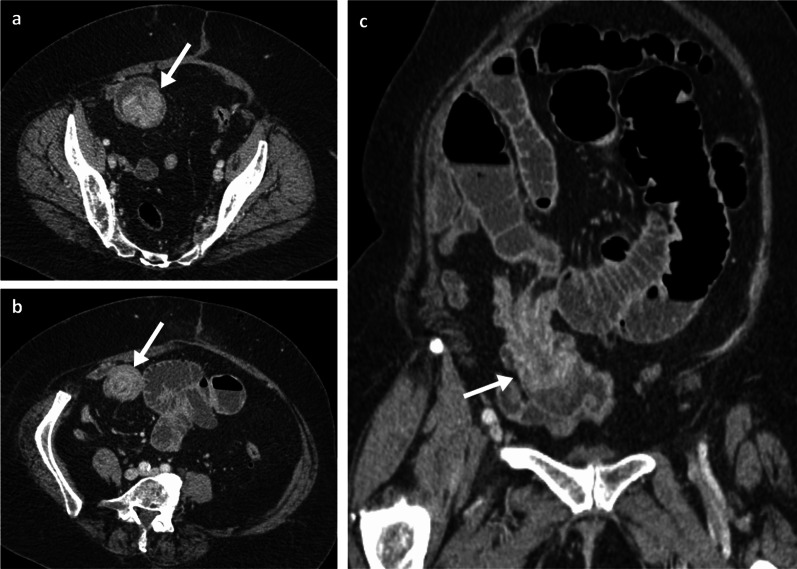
Portal phase contrast-enhanced CT axial (a, b) and oblique coronal (c) of a 71-year-old female patient with ileal melanoma metastasis with resulting obstructing intussusception (arrows)
